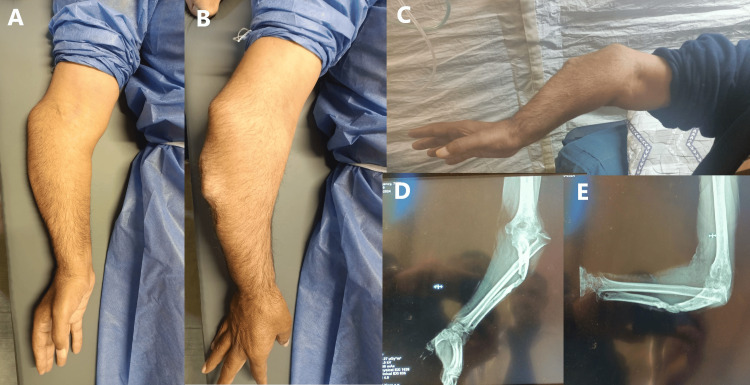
(A, B, C) Preoperative clinical images showing significant deformity, including the prominence of the dislocated radial head and the deformed ulna. (D, E) Anteroposterior and lateral forearm X-rays demonstrate a dislocated radial head, ulnar deformity with bowing, and bone loss. The imaging views are suboptimal due to the limited range of forearm movement.
